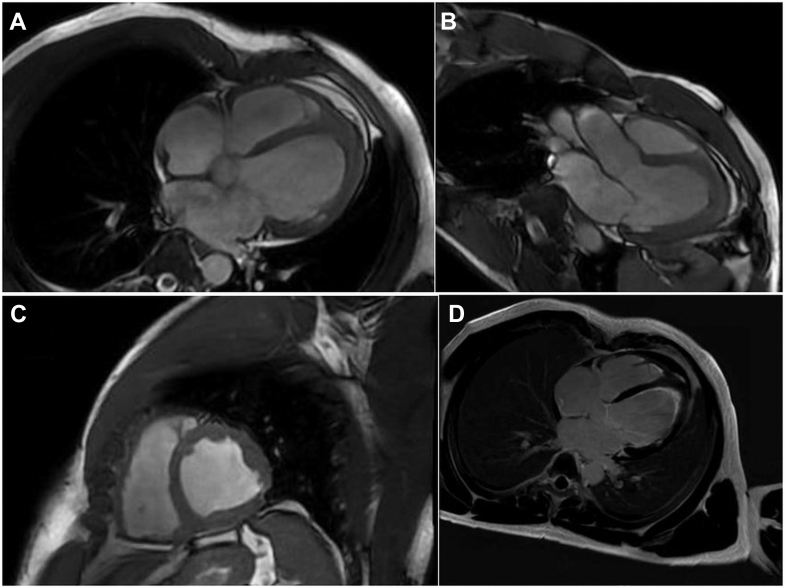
Follow-Up Cardiac Magnetic Resonance After Imatinib (A to C) Cine SSFP, apical 4-chamber (A), apical 3-chamber (B), and short-axis (C) views: nondilated left ventricle (LV) with residual apical thickening/obliteration; decrease in the size of the LV apical thrombus compared with the initial study. (D) Late gadolinium enhancement (apical 4-chamber): circumferential subendocardial enhancement at the apical level bilaterally (LV and right ventricle); additional subendocardial enhancement in the basal and midlateral LV wall, consistent with endomyocardial fibrosis. The LV apical thrombus appears laminar and smaller, without enhancement. SSFP = steady-state free precession.
